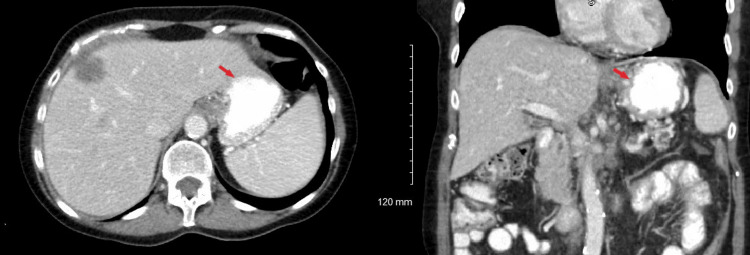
Contrast-enhanced CT abdomen and pelvis These images demonstrate a possible mass in the body of the pancreas, with a dilated distal pancreatic duct. The findings are suspicious for malignancy. Thrombosis of the splenic vein, with collateral formation, is also noted (arrows). CT: computed tomography
